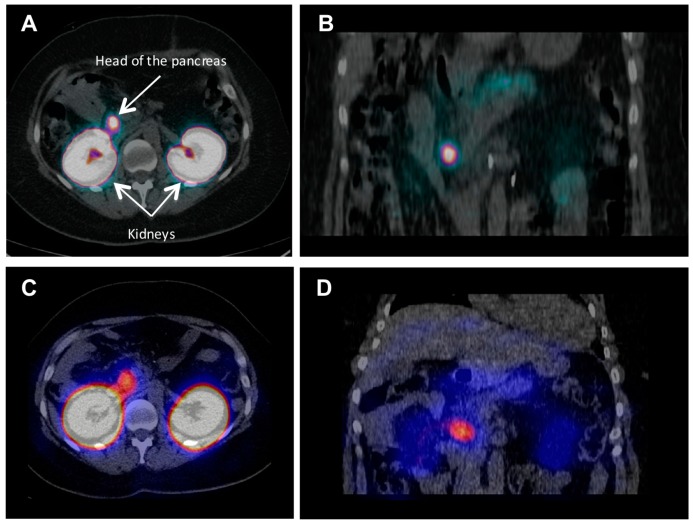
(A) Transaxial and (B) Coronal PET/CT images obtained 2.5 h after injection of 80 MBq [Nle^14^,Lys^4^^0^(Ahx-DOTA-^68^Ga)NH_2_]-exendin-4; (C) Transaxial and (D) Coronal SPECT/CT images of the same patient obtained 72 h after injection of 92 MBq [Nle^14^,Lys^4^^0^(Ahx-DOTA-^111^In)NH_2_]-exendin-4. Focal uptake is seen in the head of the pancreas. Courtesy of Dr. Kwadwo Antwi, University Hospital of Basel, Switzerland.
